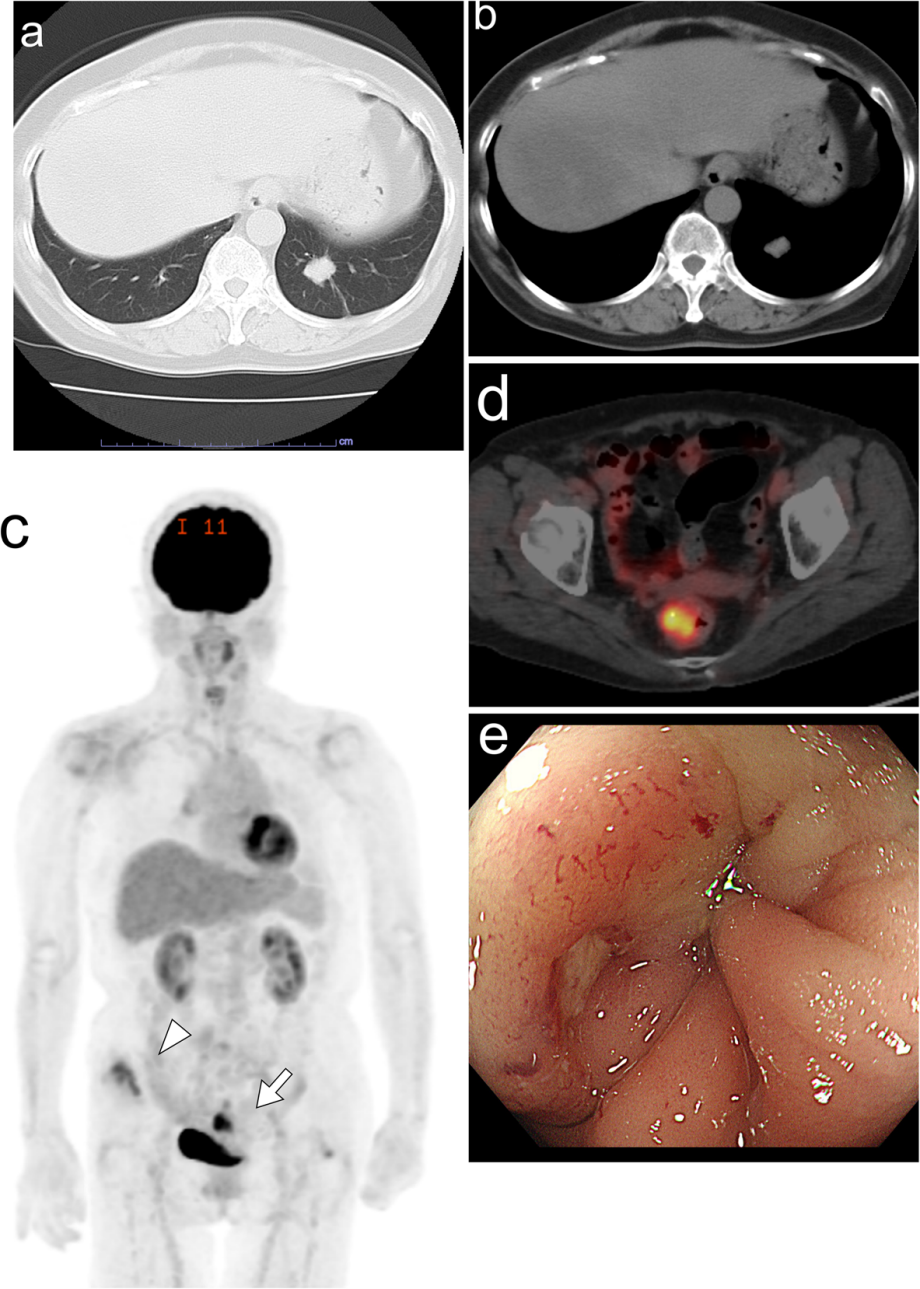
Images obtained over the clinical course. Chest computed tomography leading up to the initial diagnosis of primary pulmonary adenocarcinoma revealed a 2-cm nodule in the left lower lung lobe, as seen in the a lung window image and b mediastinal window image. Positron emission tomography leading to the diagnosis of rectal metastasis showed an accumulation of ^18^F-fluorodeoxyglucose in the upper rectum (arrow) and right iliac bone (arrowhead), as seen in c the whole body image and d horizontal section. Colonoscopy revealed e an intramural rectal mass with central ulceration
